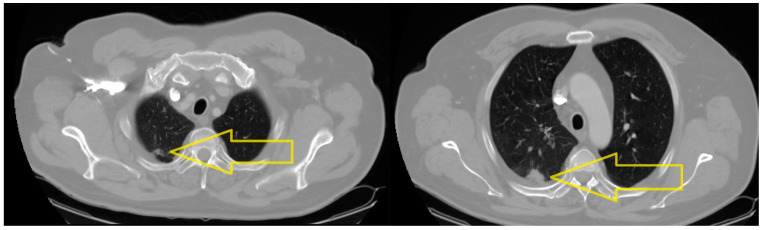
CT scan chest (October 2022): An expanding nodular mass in the apical segment of the right upper lobe with maximum axial diameters of 1.7/0.7. An expanding nodular mass plated in the posterior pleura of the posterior segment of the right upper lobe with maximum axial diameters of 2.2/1.5 mm.
